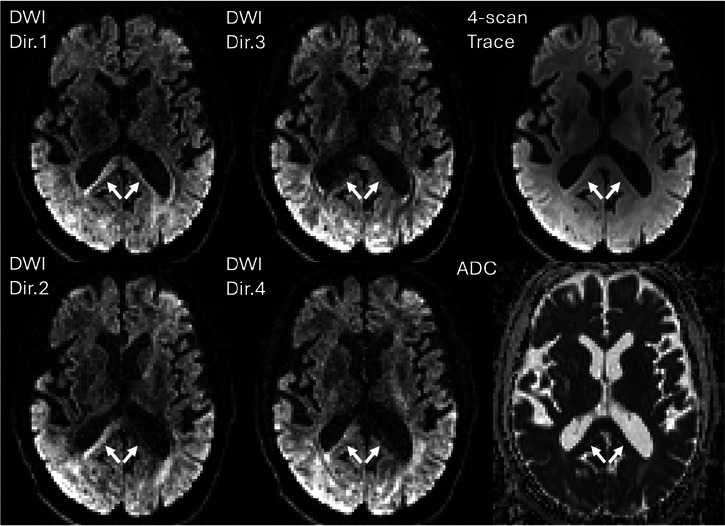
Exemplar 4-scan trace DWI data. DWI images are acquired at a given *b*-value (here 1000 s/mm^2^) with diffusion gradients oriented along four different directions (DWI Dir.1-4). The mean DWI image (4-scan trace) is then computed by averaging the DWI images along the four directions, and an ADC map is estimated from it. Arrows indicate signal changes due to tissue anisotropy, mitigated in the 4-scan trace and the corresponding ADC map
